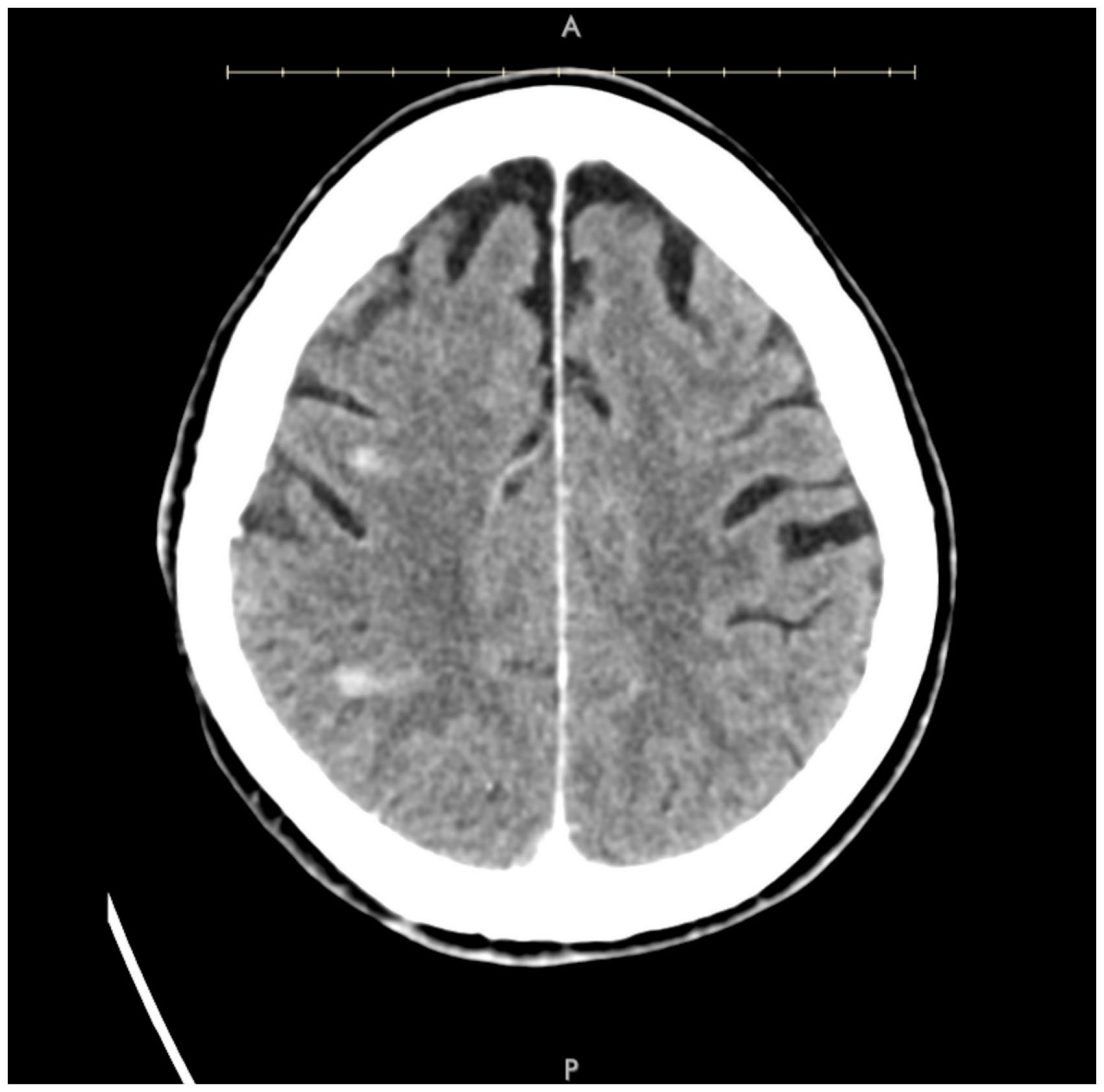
Axial post-contrast CT demonstrating small areas of enhancement within the right MCA territory.
